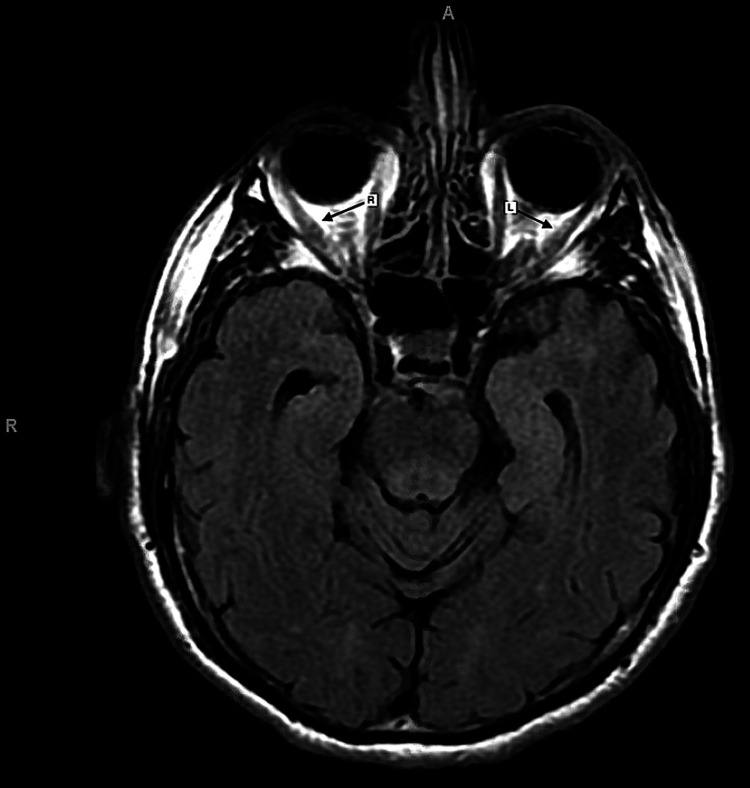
MRI of the orbits depicting lateral rectus muscle belly enhancement "L": Arrow to the left lateral rectus muscle; "R": Arrow to the right lateral rectus muscle
